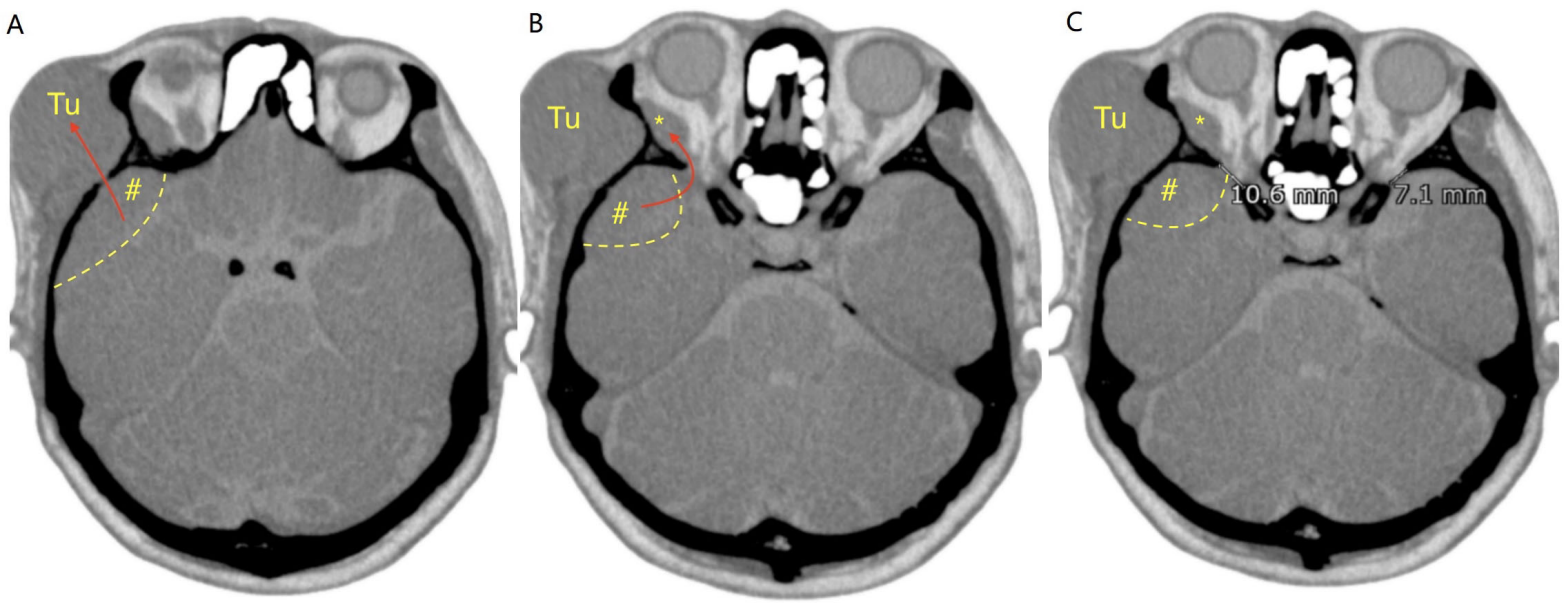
Preoperative bone-window CT demonstrating inferred routes of extension. (A) Axial bone-window CT showing a lesion centered at the floor of the middle cranial fossa (#); the long arrow indicates a suspected transosseous/foraminal route toward the extracranial temporal compartment. (B) Tumor extension into the orbit through an enlarged superior orbital fissure (arrow). (C) Right-to-left asymmetry with enlargement of the right superior orbital fissure. *, intraorbital lesion; #, middle cranial fossa lesion.
